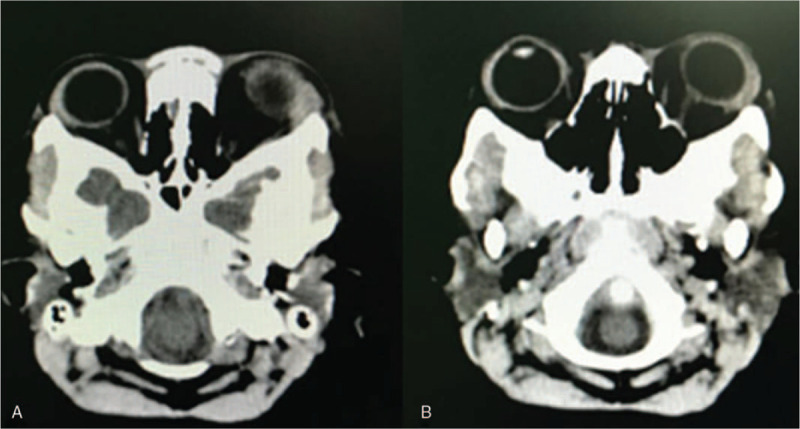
Postoperative computed tomography scan. (A) and (B) Complete resolution of the hematoma.
